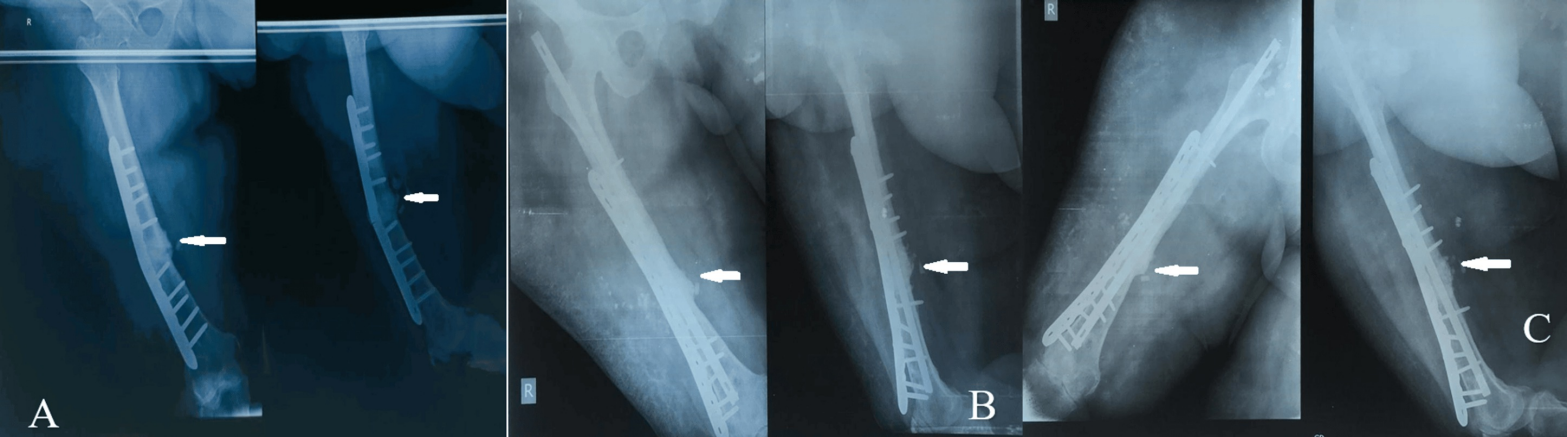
A 36-year-old male with implant breakage and nonunion of the right femur shaft fracture. A. Preoperative radiograph taken nine months after initial surgery revealing implant breakage and nonunion. B. Immediate postoperative radiograph following IMN, DCP plate, and graft augmentation. C. Three months postoperative radiograph displaying bone union with robust consolidation at the fracture site in a radiograph taken three months postoperatively. IMN, intramedullary nailing; DCP, dynamic compression plate
